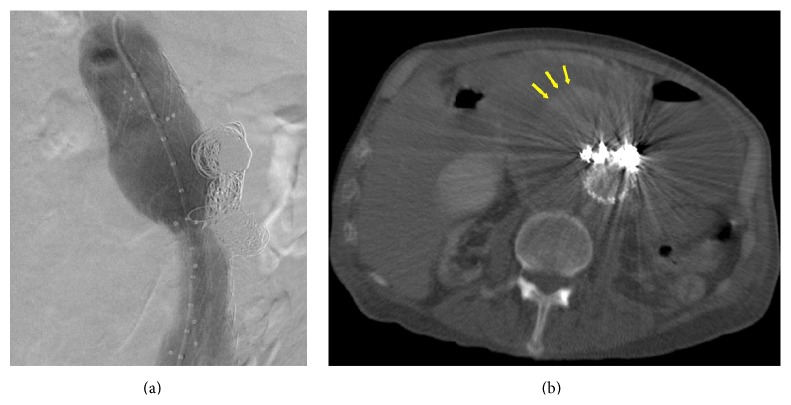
(a) Angiogram showed the complete disappearance of type 3b endoleak. (b) CT angiography showing peripheral high-density area in the sac, which could be related to fresh clot or residual contrast media during coils embolization.
